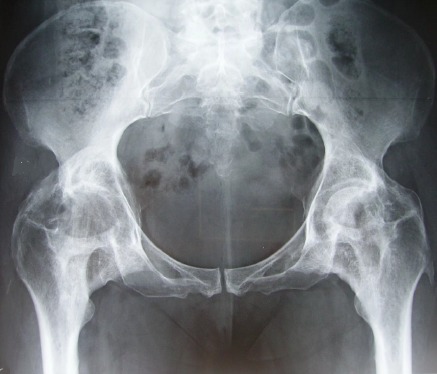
Radiographie standard du bassin de face montrant des ossifications hétérotopiques bilatérales de la hanche
